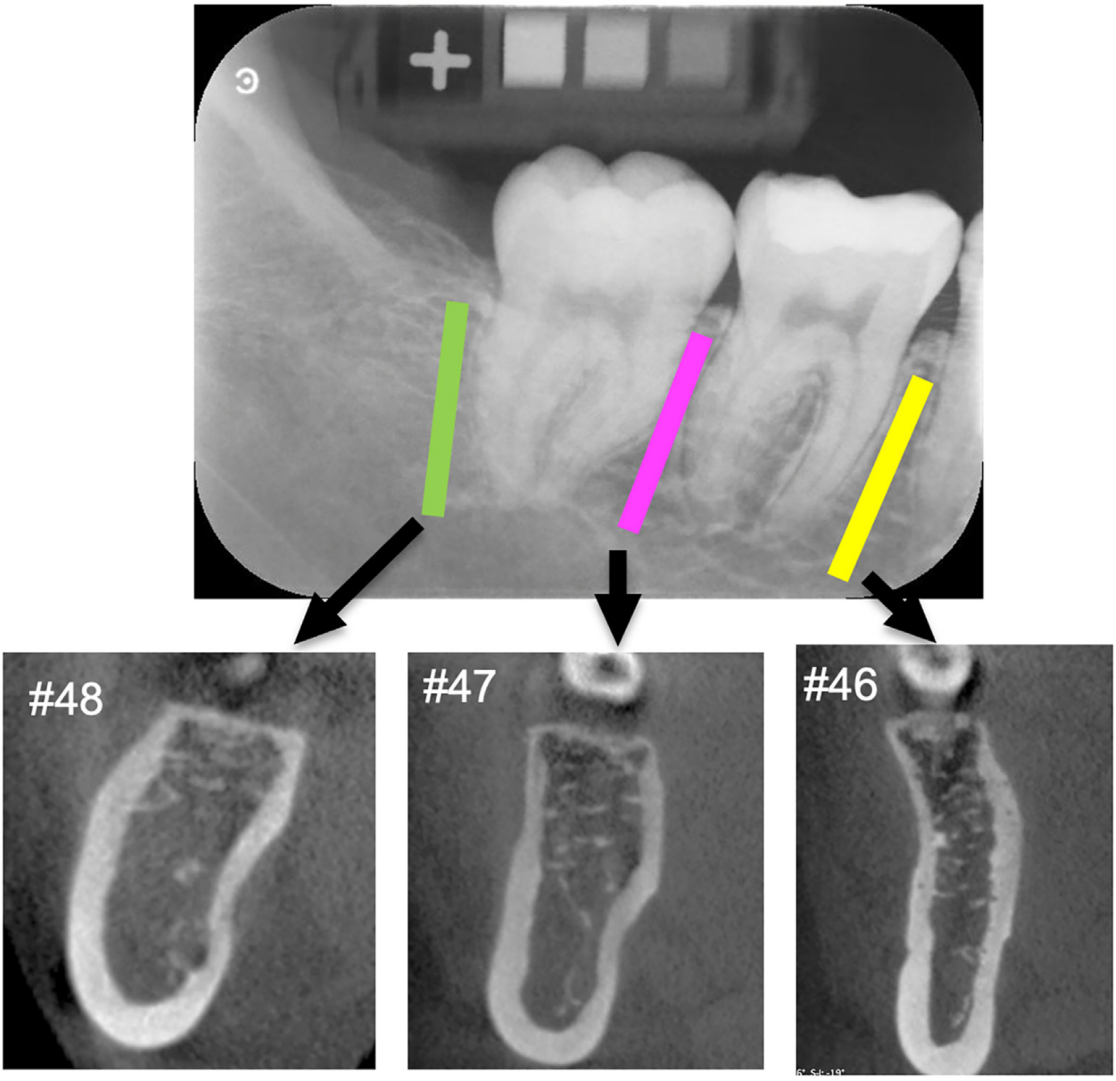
ROIs in an intraoral X-ray image and multi-planer reconstruction CBCT image of the same region. Three ROIs were set in an intraoral X-ray image to measure BMD. Cross sectional CBCT images were reconstructed in the tooth number 46, 17, and 48 regions.
